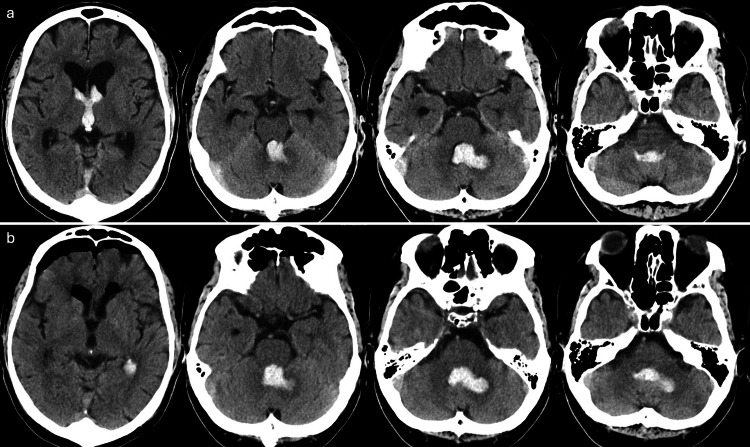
CT imaging of head at onset and postoperatively. a: This is a CT image taken at onset, showing a hematoma in the left cerebellum. The hematoma has ruptured into the ventricular system, with blood also present in the third ventricle.
